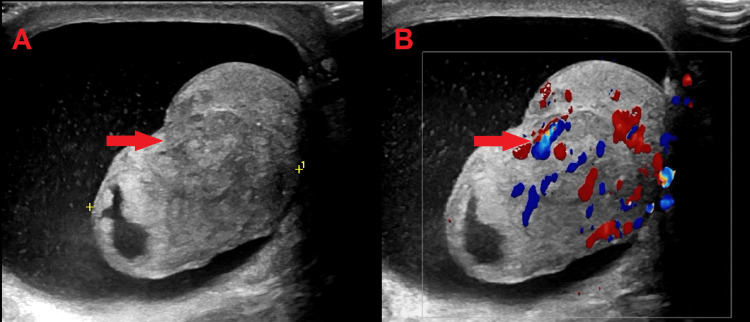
Ultrasound and color Doppler imaging of left testicular lesion. (A) Grayscale image depicting a 2.4-cm well-encapsulated, hyperechoic intratesticular mass (red arrow) highly suspicious for malignancy. (B) Color Doppler image showing significant vascular flow within the lesion (red arrow)
